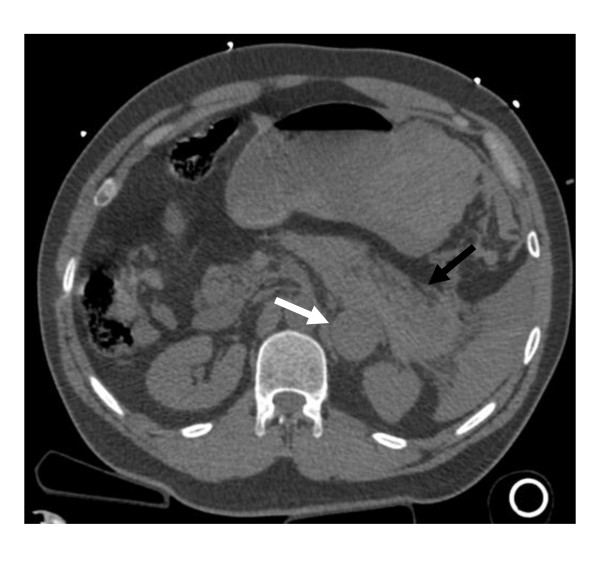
CT scan of the abdomen with left adrenal mass (white arrow) and associated intra-peritoneal hemorrhage (black arrow) obtained on presentation to the outside hospital.
